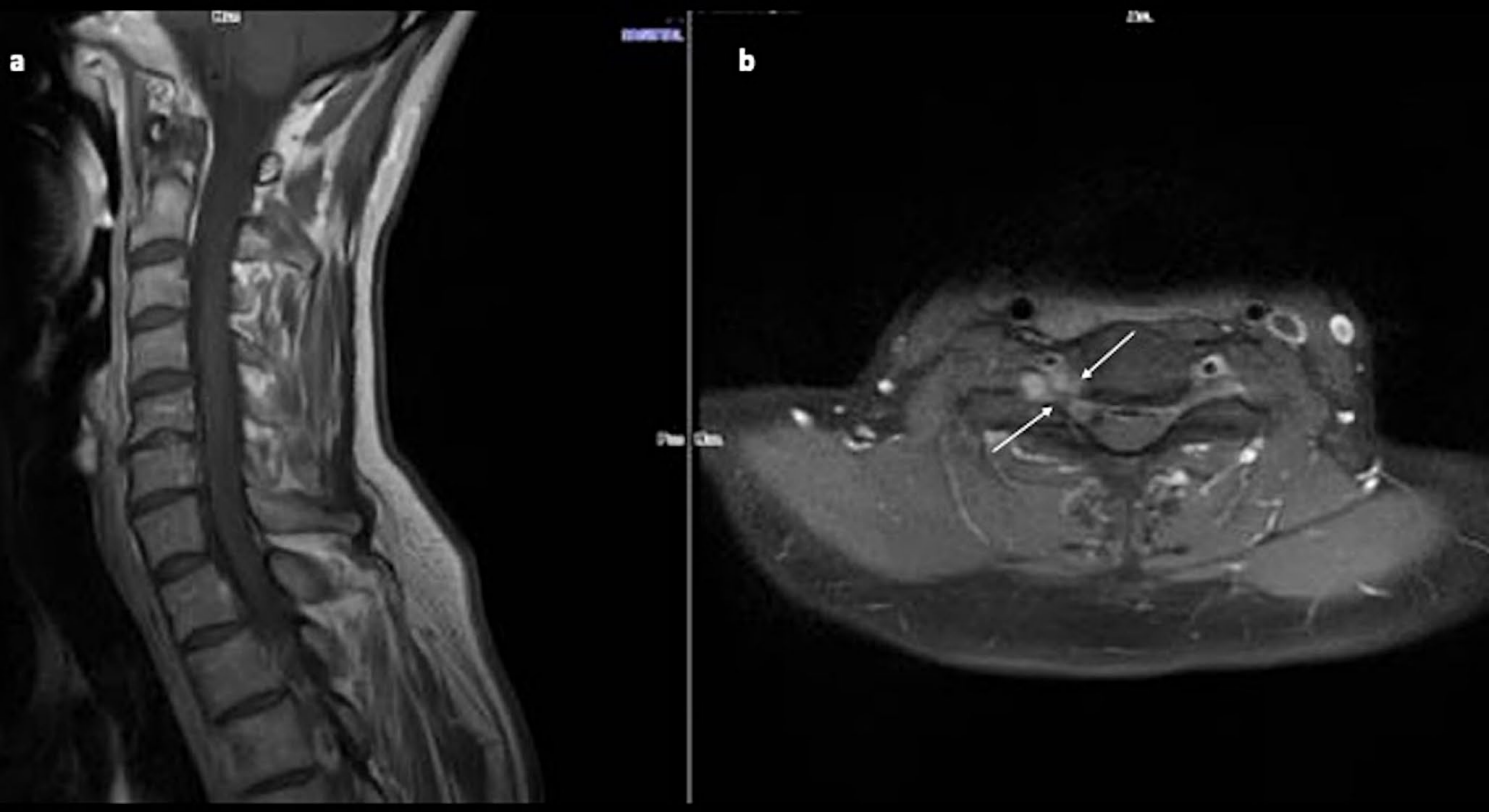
Follow-up cervical MRI with contrast-enhanced T1 sequences (Panel a sagittal, Panel b axial views) showing absence of tumor recurrence at the C6-C7 right foramen (white arrows)
